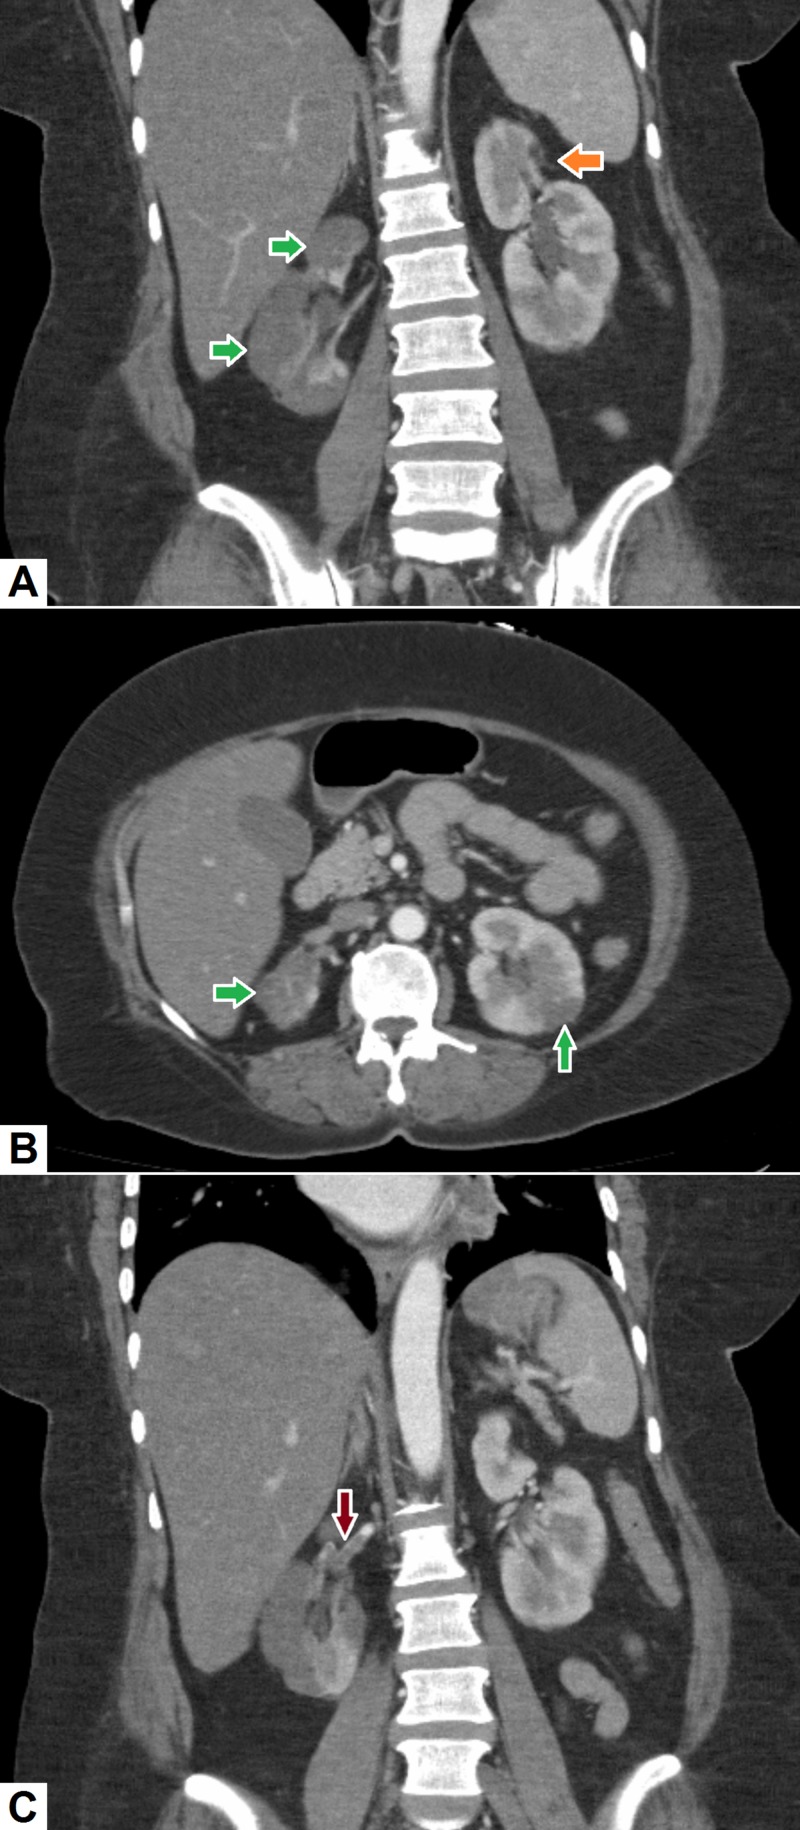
Renal infarction. (A) Coronal and (B) axial computed tomography (CT) sections demonstrate multiple low-density areas (green arrows) in the periphery of both kidneys representing infarcts. An area of focal scarring (orange arrow) can also be seen at the upper pole of left kidney on the coronal section. (C) Coronal image demonstrates a filling defect (red arrow) in the right renal artery representing a thrombus.
